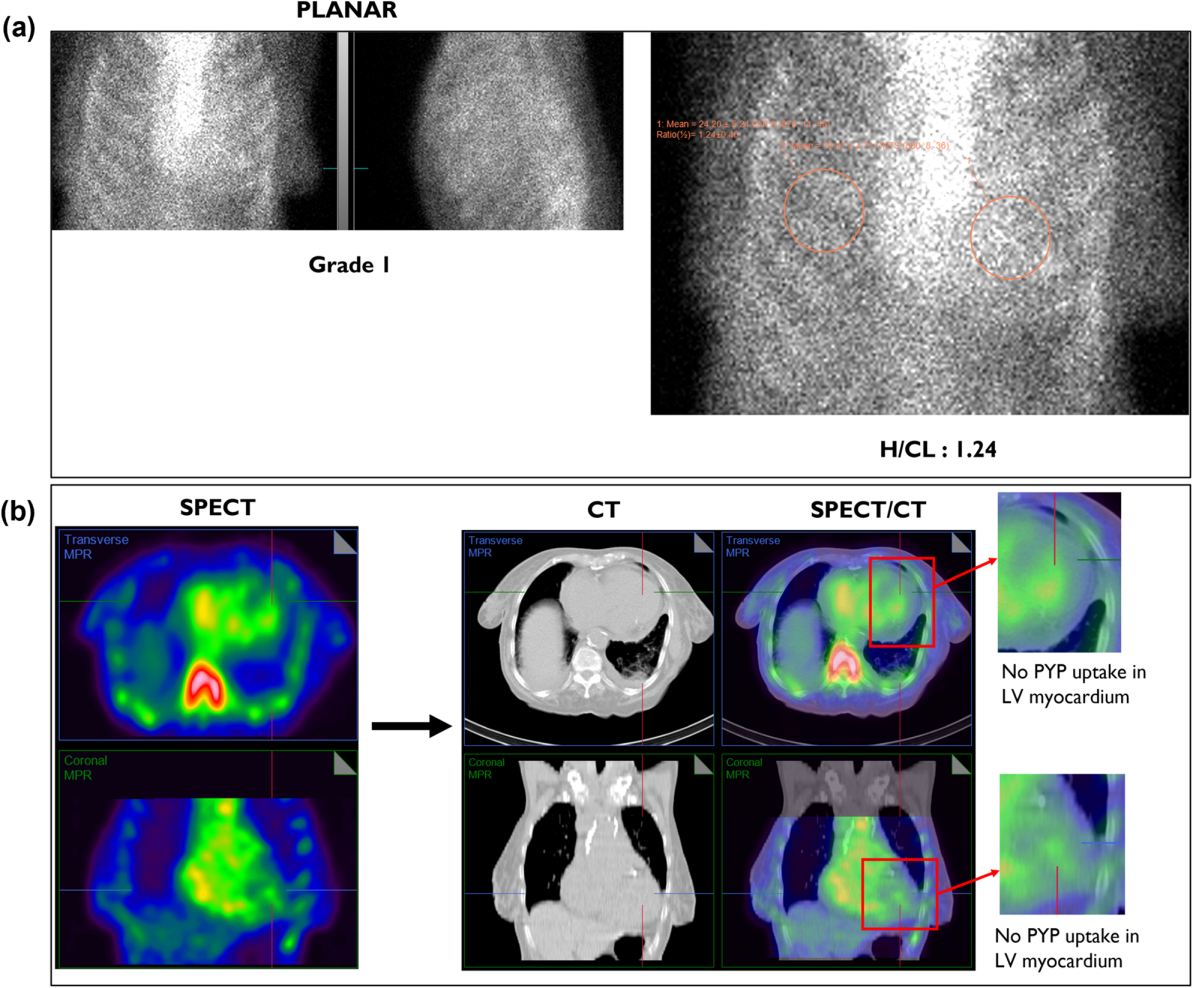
(A) Three-hour planar imaging shows Grade 1 visual tracer uptake and H/CL ratio of 1.24; interpreted as “equivocal for ATTR amyloidosis”.^5^ Visual assessment of planar images further demonstrates relatively less myocardial uptake compared to the ribs. (B) SPECT alone demonstrates an empty looking LV cavity, which is shown to be the thickened septal wall on the fused CT images. Also note that the inferior lateral border of the LV wall is not demonstrating any PYP uptake with SPECT/CT fusion. SPECT/CT reclassifies the case from equivocal to “not suggestive”
